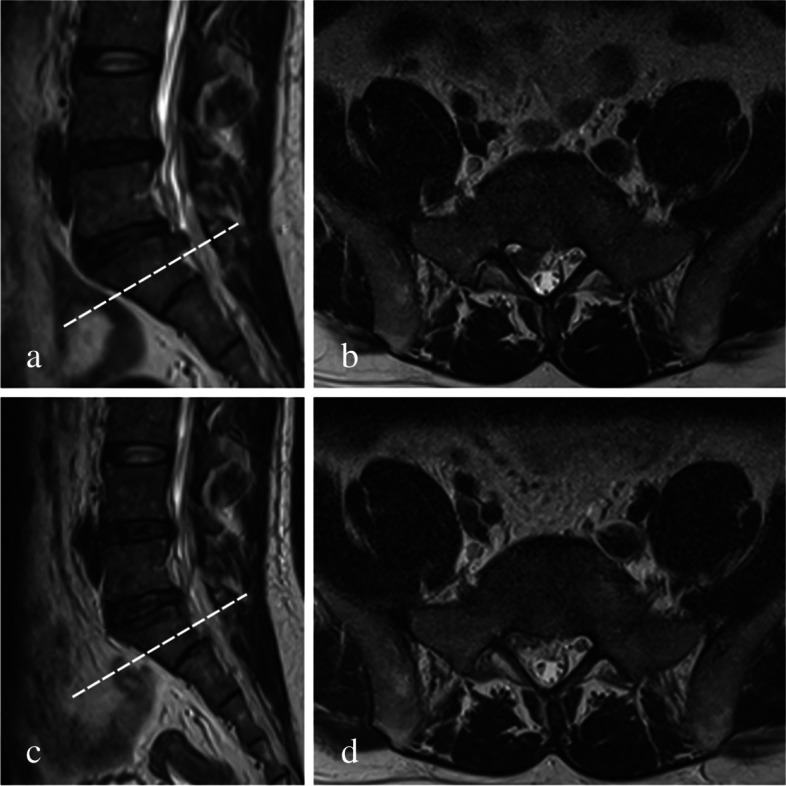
a Preoperative sagittal and (b) axial T2 MRI showing a highly down-migrated disc from L5 to S1. c Postoperative sagittal and (d) axial T2 MRI showing successful surgical removal of the downward migrated fragments
